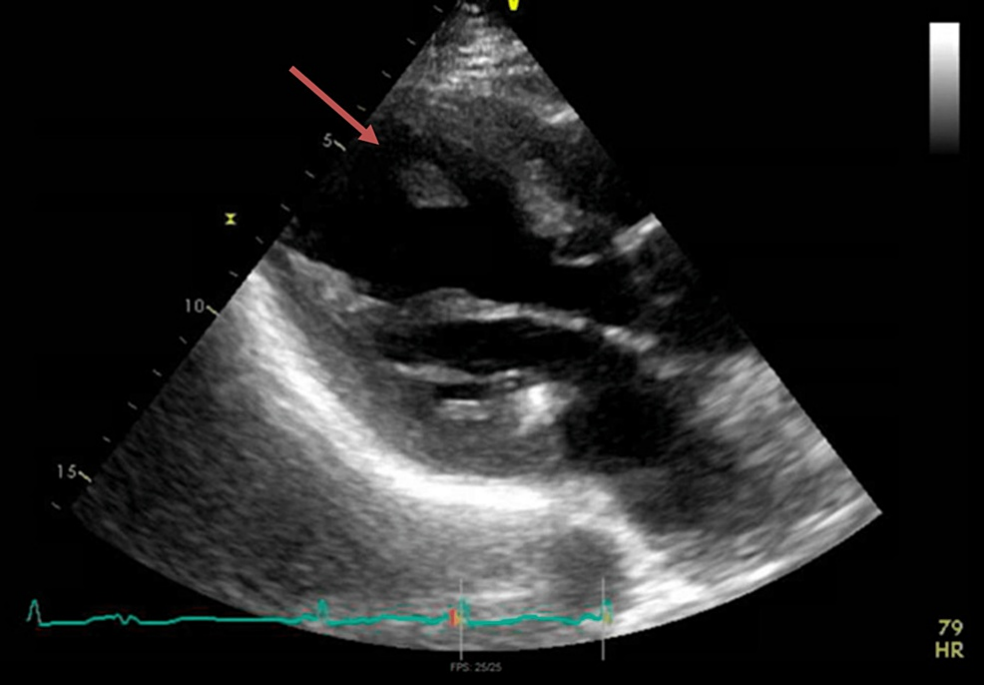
The left ventricle with a thrombus attached by a thin fibrinous string as shown by the red arrow on the echocardiogram parasternal long axis.
